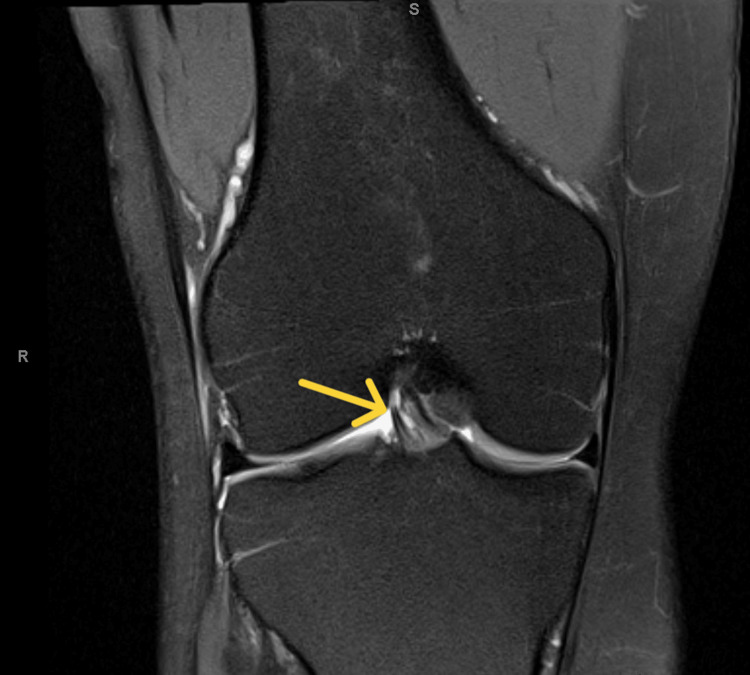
AP MRI of ACL exhibiting 10% tear AP, anterioposterior; ACL, anterior cruciate ligament; MRI, magnetic resonance imaging
